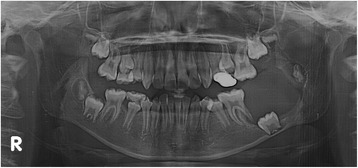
A panoramic radiograph before marsupialization at the initial visit revealing a well-circumscribed, unilocular radiolucency in the region of the left mandibular molars, with an unerupted second molar and dental follicle of the third molar
